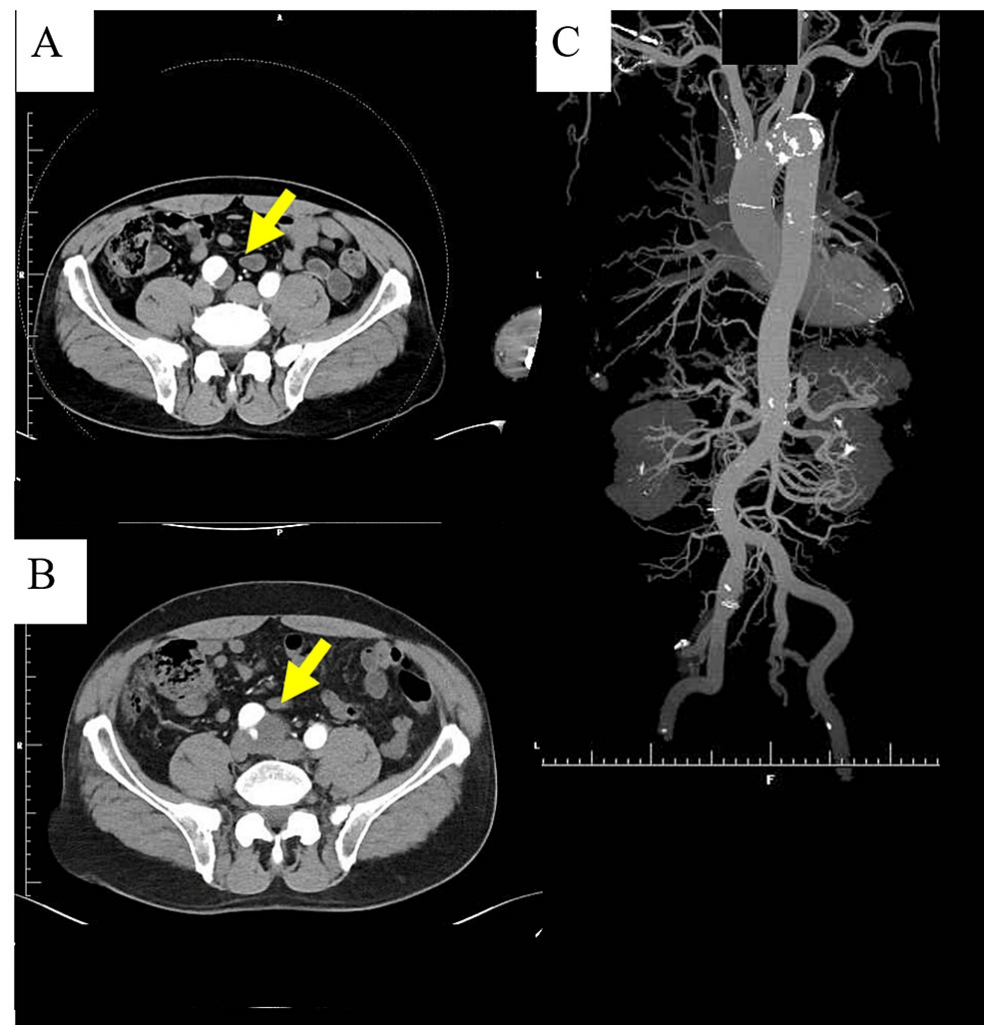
A series of computed tomography angiography images. A. The iliac artery aneurysm four years ago (an arrow). B. The iliac artery aneurysm on admission (an arrow). C. Three-dimensional reconstruction of the aorta. Calcification was found predominantly in the aortic arch.
